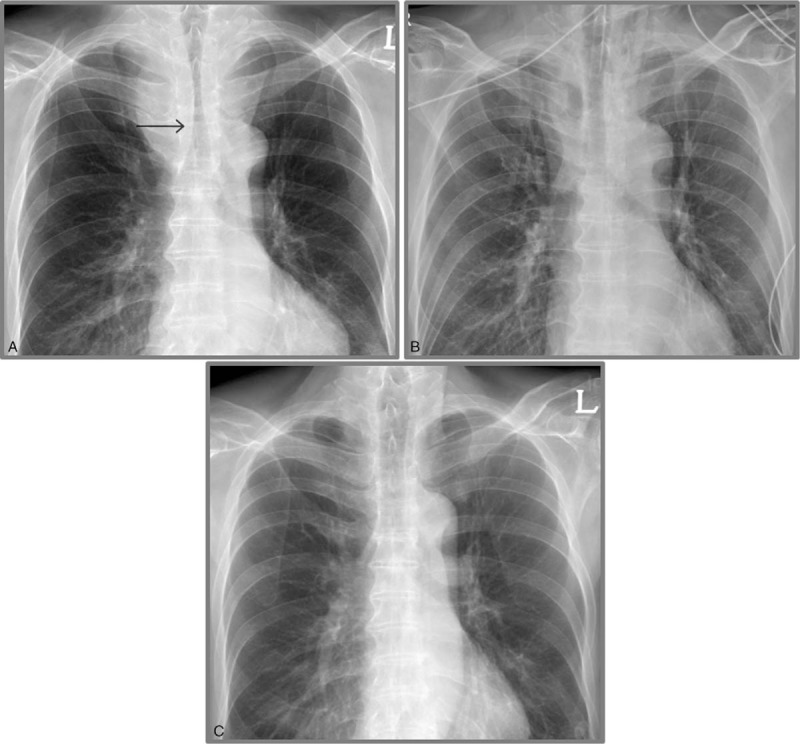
Perioperative chest radiographs for an elderly patient with a massive intrathoracic goiter causing severe tracheal obstruction with positional symptoms. The patient received extracorporeal membrane oxygenation before anesthesia induction. The tip of the endotracheal tube is placed at a depth of 28 cm (arrow line) beyond the narrowest portion of the trachea (A). After the surgical procedure, the endotracheal tube is exchanged with a silastic cuffed tube, which is fixed at a depth of 23 cm (B). A postoperative chest radiograph shows restoration of the tracheal lumen after extubation (C).
